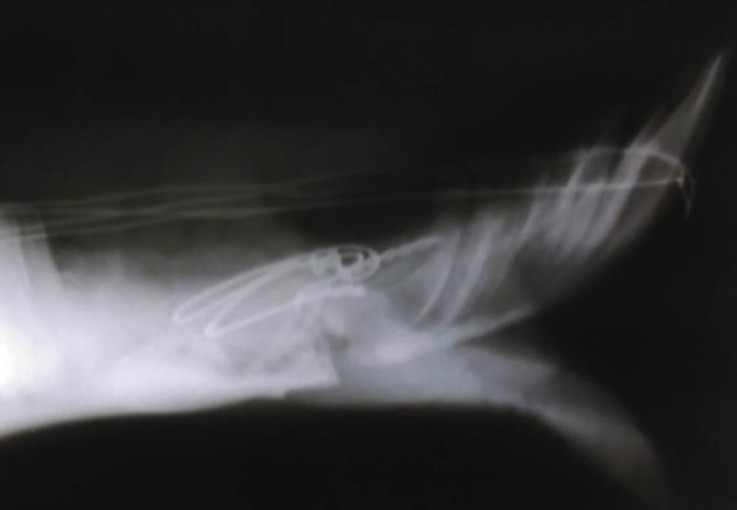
Postoperative radiography of repaired mandibular fracture using orthopedic wire.
